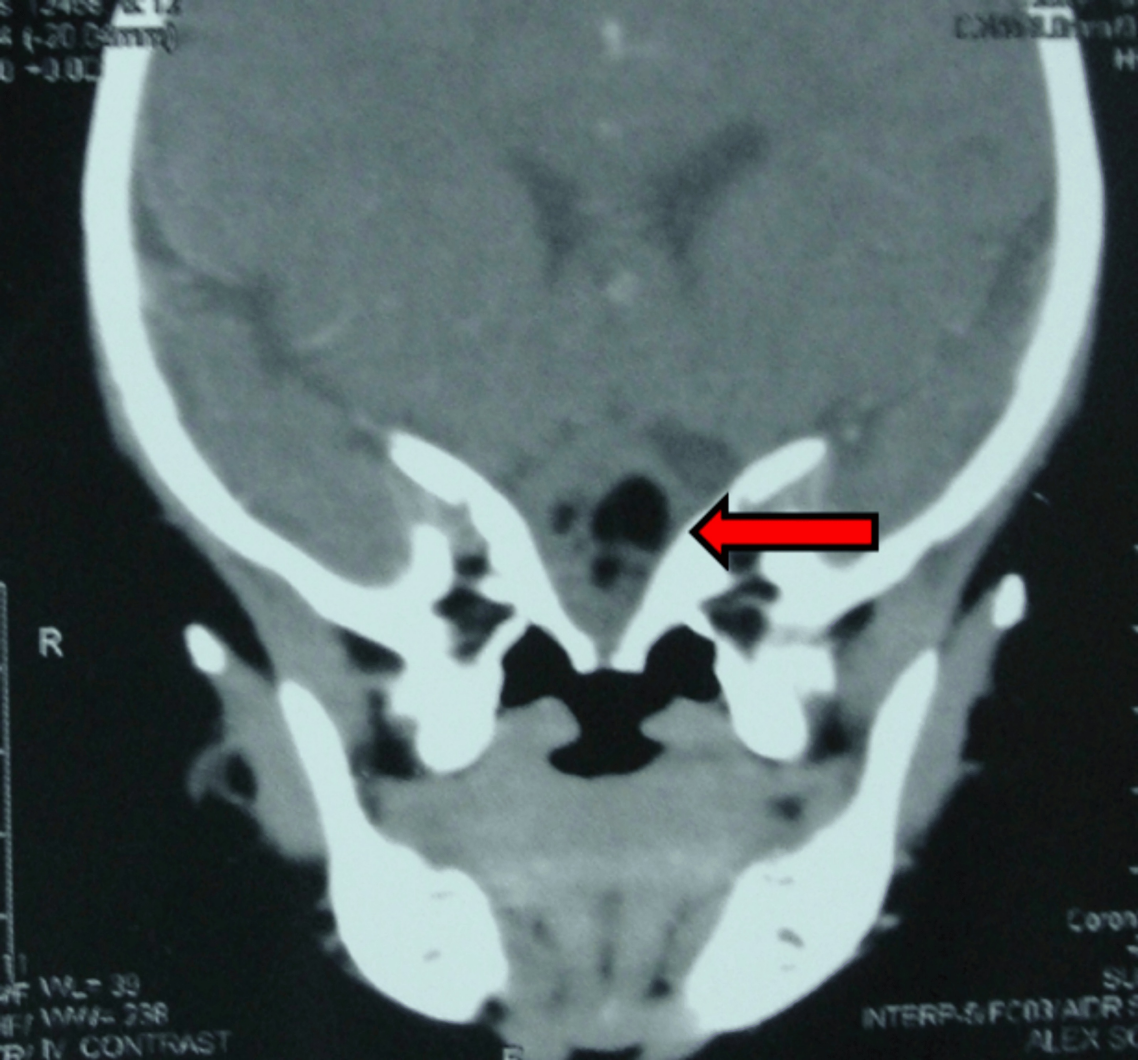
Axial CT scan showing a heterogeneous fat-density mass (red arrow) at the roof of the oral cavity, measuring 2.7 × 2.6 × 2.3 cm, with internal calcifications, communicating through an anterior skull base defect with an intracranial component of similar attenuation. CT: computerized tomography
